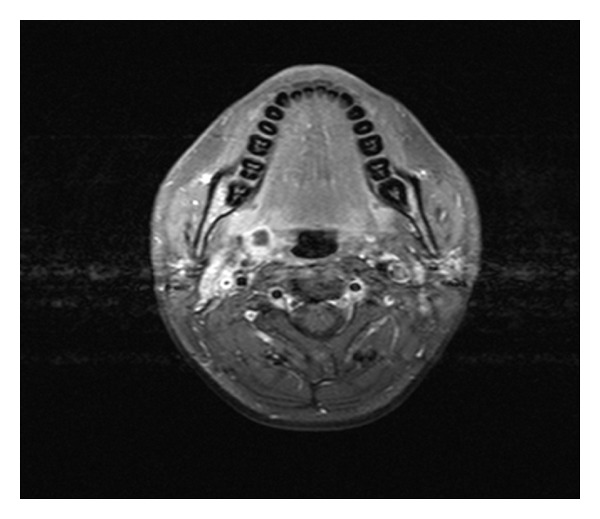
MRI head shows an enhancing structure, an abscess on the right side measured 1.3 × 1.5 × 1.3 cm, and also a DVT in the IJ vein more posterior and lateral to the abscess.
